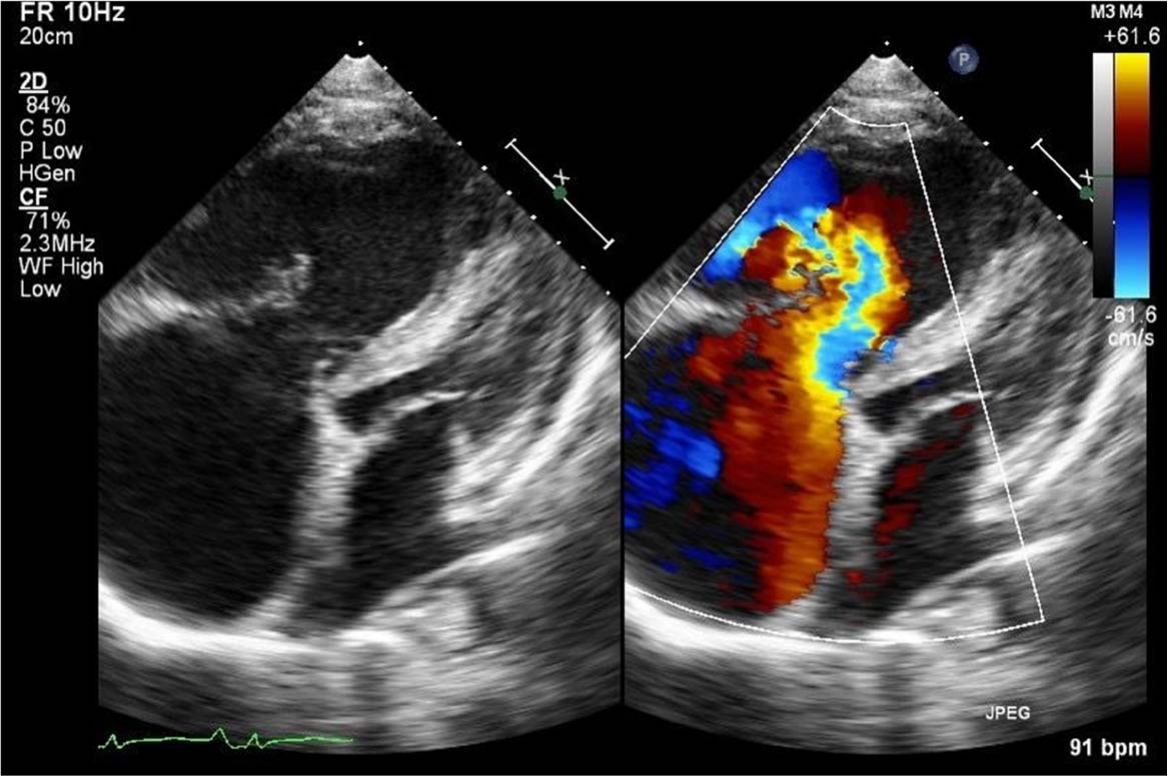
Echocardiographic findings revealing severe dilation of the main right ventricle (RV) and RV outflow tract, significant RV hypertrophy, severely reduced RV systolic function, severe right atrial dilation, severe tricuspid regurgitation, and elevated central pressures.
